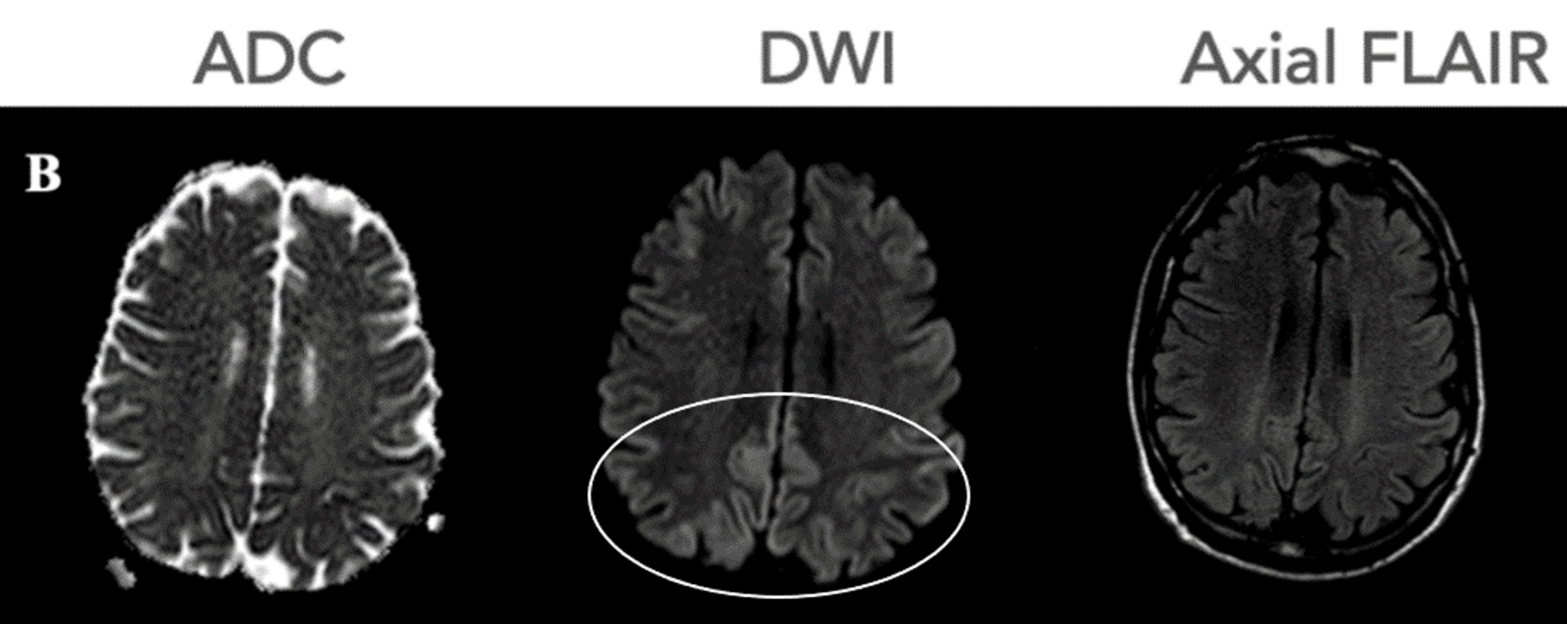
A second brain magnetic resonance imaging (MRI) performed seven days after the initial scan showed more extensive cortical hyperintensities on DWI (circle). ADC: apparent diffusion coefficient map; DWI: diffusion-weighted image; FLAIR: fluid attenuated inversion recovery
